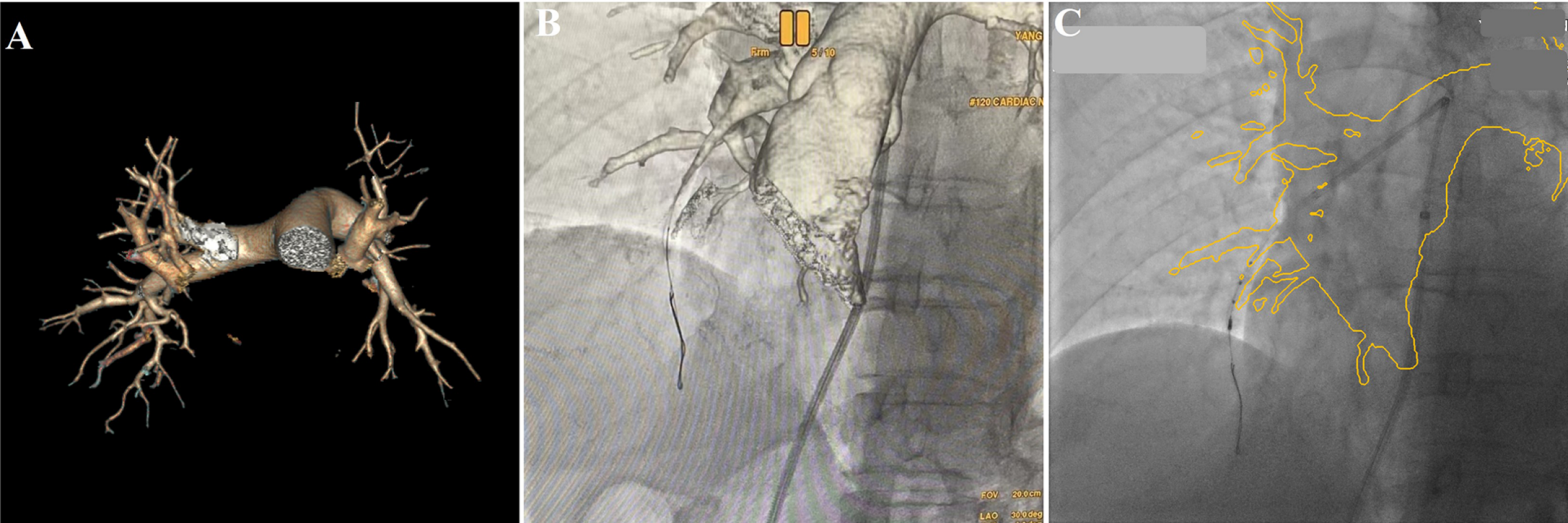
Virtual reality reconstruction of the patient's pulmonary artery based on previous pulmonary artery CTA image (A), real-time image fusion navigation guided the direction of the guidewire via pulmonary artery to A7 (B, Virtual reality), and guided the IUVS into A8a (C, outline volume-rendered) during BPA procedure.
